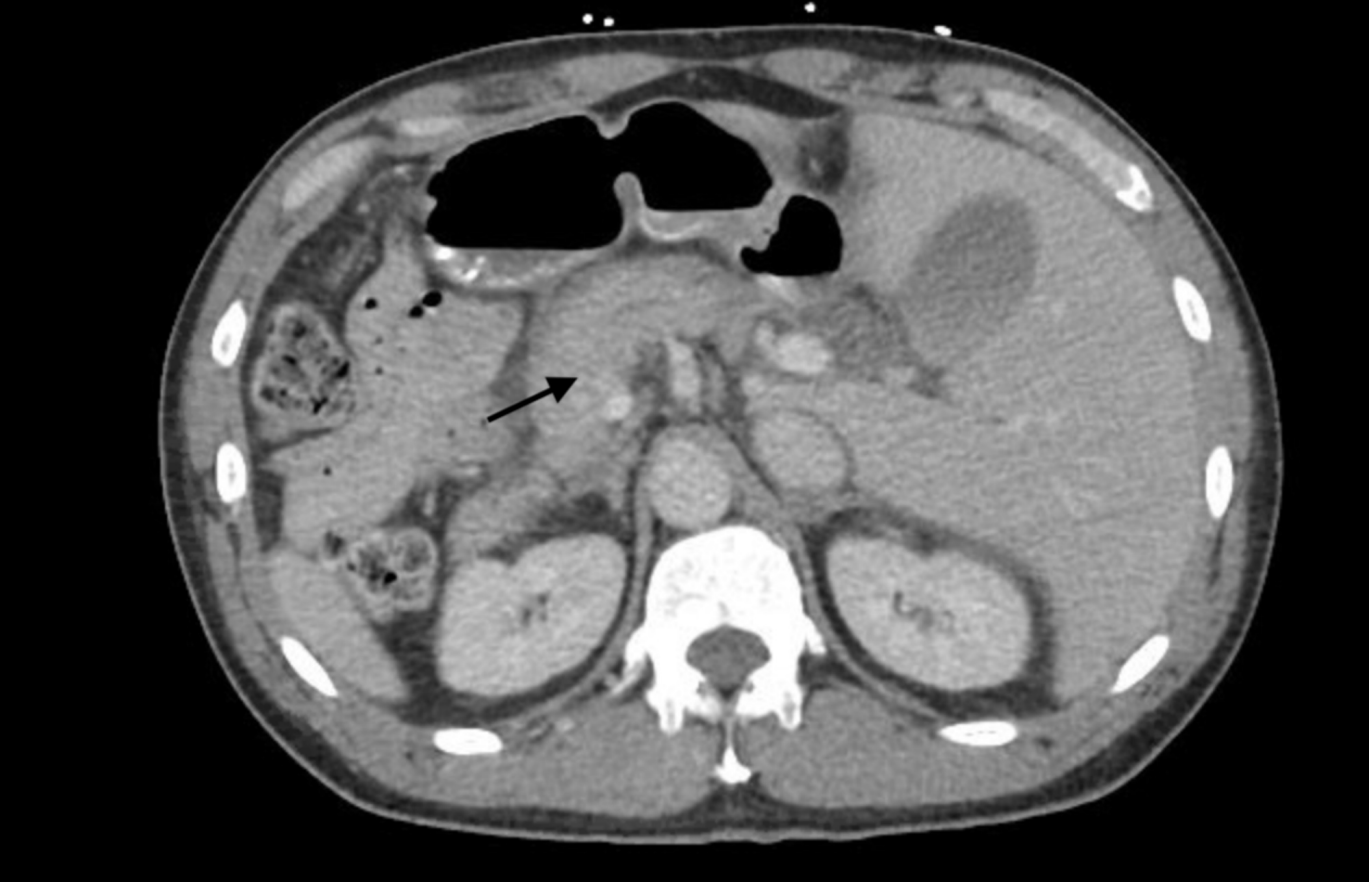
Computed tomography of the abdomen showing heterogenous pancreas with lack of contrast enhancement (arrow)
